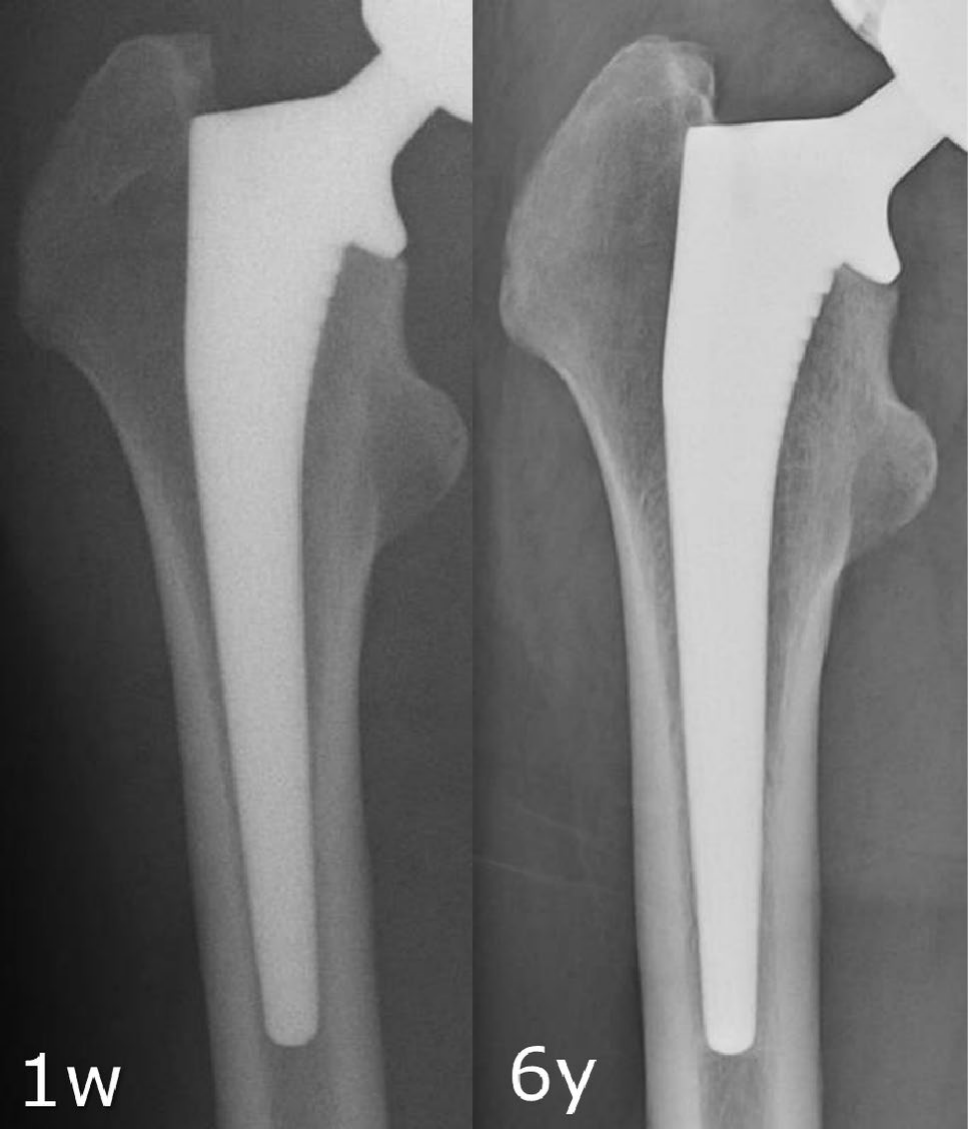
Longitudinal hip radiographs of a 55-year-old male patient who had THA using CORAIL^®^ collared stem with bone graft showing no evidence of reactive lines or stress shielding, 6 years after surgery
